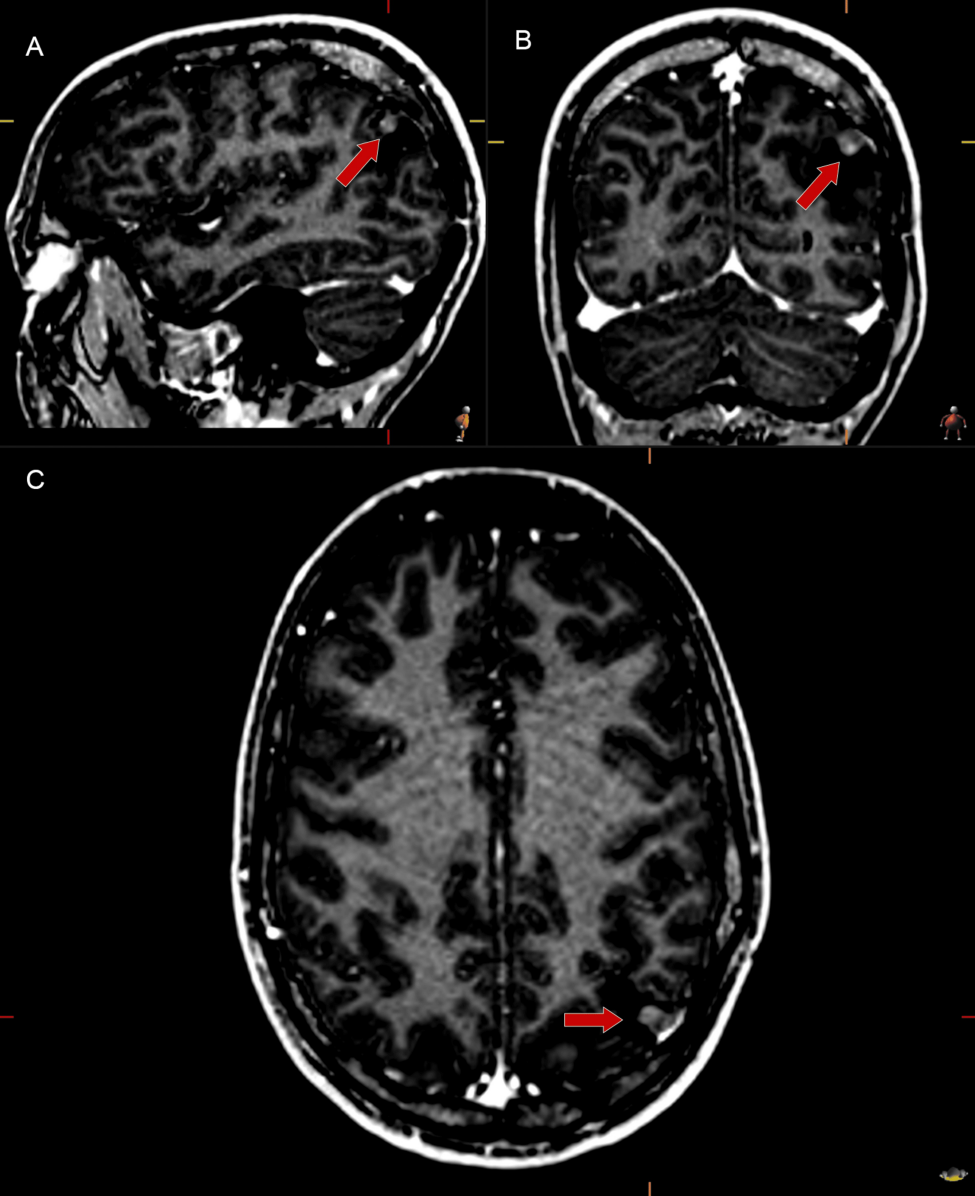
Post-gadolinium contrast-enhanced MRI (sagittal, coronal, axial) showing focal enhancement consistent with recurrent left parietal ganglioglioma (arrows) Post-gadolinium contrast-enhanced brain magnetic resonance imaging (A sagittal, B coronal, and C axial views) demonstrates focal areas of enhancement in the posterior superior aspect of the previous surgical cavity, consistent with recurrent left parietal ganglioglioma (arrows)
